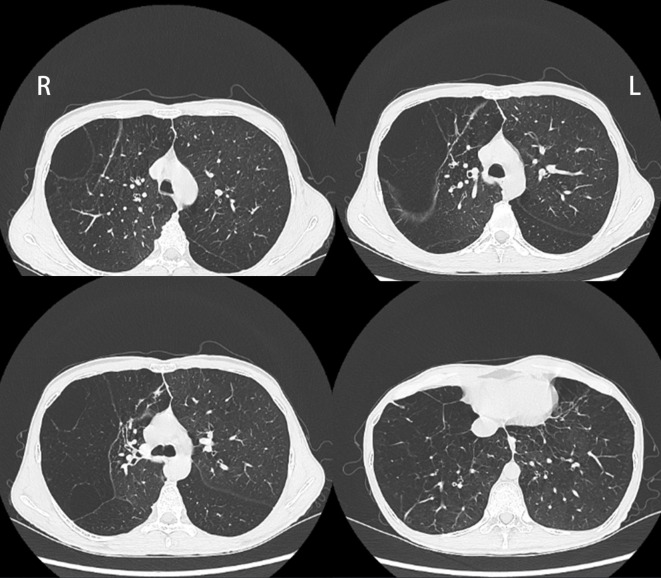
Chest CT showed bilateral emphysema and pulmonary bullae.
